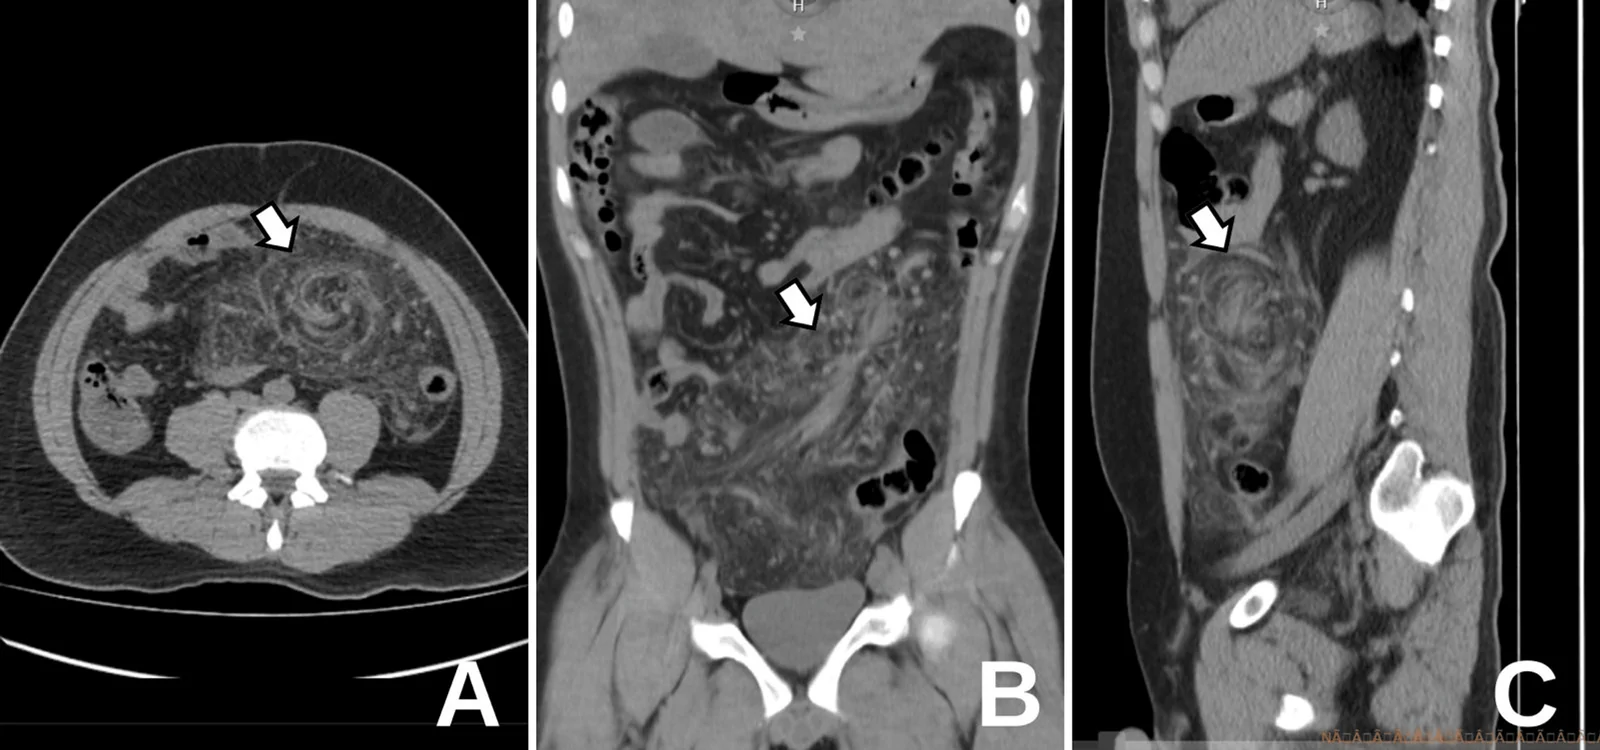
Abdominopelvic Computed Tomography in Non-contrast Slices Abdominal computed tomography in axial non-contrast slices (A), coronal reconstruction (B), and sagittal reconstruction (C) demonstrating increased density of the omental fat with a concentric and helical stranding pattern, forming the "whirl sign." Thickened vascular structures are observed converging toward a central point corresponding to the twisted vascular pedicle, associated with inflammatory changes in the adjacent adipose tissue. Findings are compatible with torsion of the greater omentum
